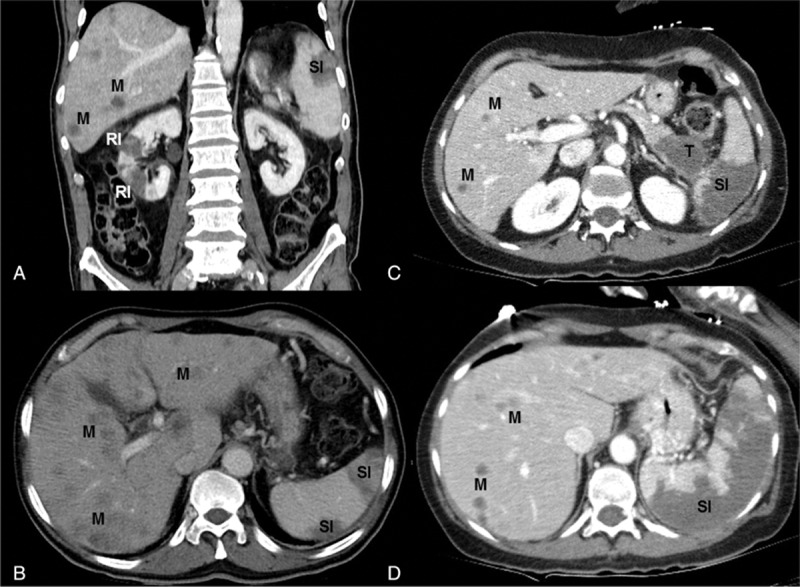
Abdominal CT scan showing pancreatic cancer (T), hepatic metastasis (M), renal (RI), and splenic infarctions (SI). A, B, CT scan without contrast. C, D, Contrast-enhanced CT scan. CT = computed tomography.
